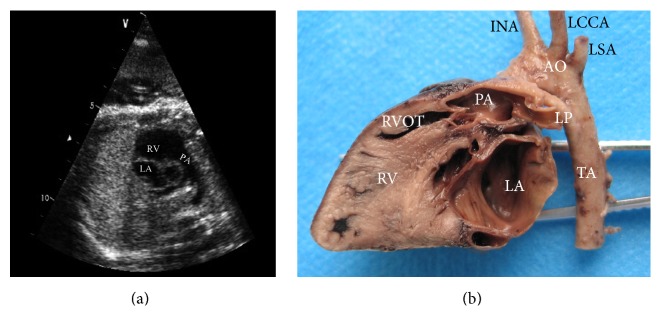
Tetralogy of Fallot (TOF) (28 w). (a) The right ventricular outflow tract echocardiography section. (b) The right ventricular outflow tract sectional anatomy. INA: innominate artery; LCCA: left common carotid artery; LSA: left subclavian artery; PA: pulmonary artery; RVOT: right ventricular outflow tract; RV: right ventricle; LA: left atrium; AO: aorta; LPA: left pulmonary artery; TA: thoracic aorta.
